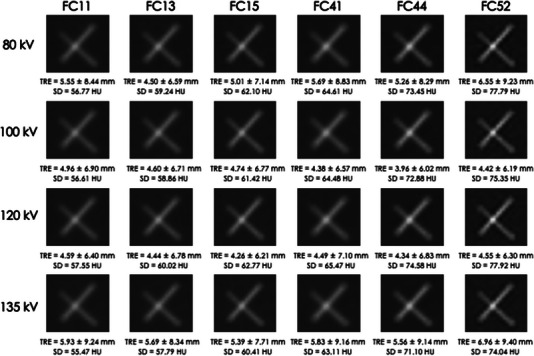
CT image obtained by different reconstruction algorithms and tube voltages.
